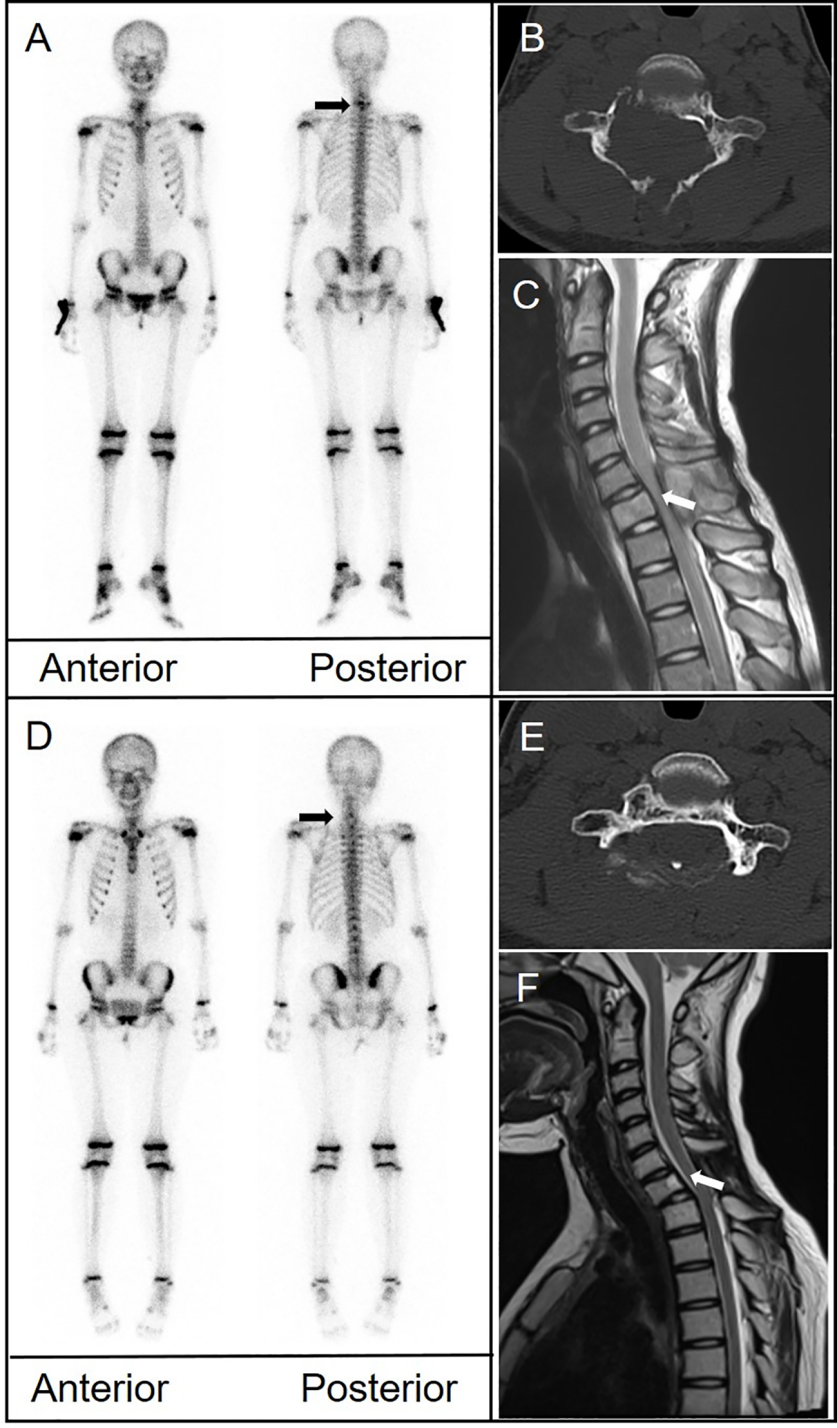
(A) Bone scintigraphy (anterior and posterior) demonstrated avid radiotracer uptake in the lower cervical spine (arrow). (B) CT depicted significant structural damage to the C7 vertebral body, right pedicle, and posterior elements. (C) Magnetic resonance imaging (MRI) showed signal change in the C7 body and posterior elements with associated spinal canal stenosis and cord compression (arrow). (D) A follow-up bone scan 11 months later showed disappearance of uptake in the cervical spine. (E) CT showed persistent bone destruction. (F) MRI showed improvement in spinal canal stenosis.
